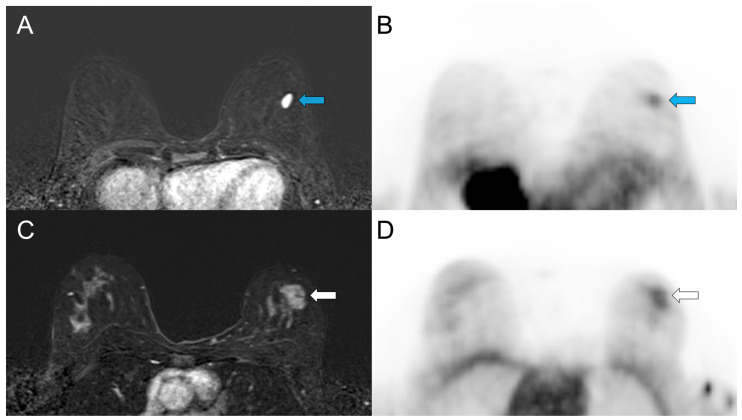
^18^F-FES PET/MRI of the breast in two patients with benign breast lesions. Subtracted dynamic postcontrast T1-weighted MR images (A,C) and corresponding ^18^F-FES PET images (B,D). (A,B) A 46-year-old woman with known fibroadenoma in the left breast stable in shape and size for more than two years (blue arrows). MRI shows oval, circumscribed, homogeneous enhancing mass. ^18^F-FES SUVmax is 1.18 and SUVmean is 0.73. (C,D) A 50-year-old woman with biopsy-proven benign fibroadenoma in left breast (white arrows). MRI shows irregular, partly indistinct mass with non-enhancing septa. ^18^F-FES SUVmax is 1.57 and SUVmean is 1.02.
